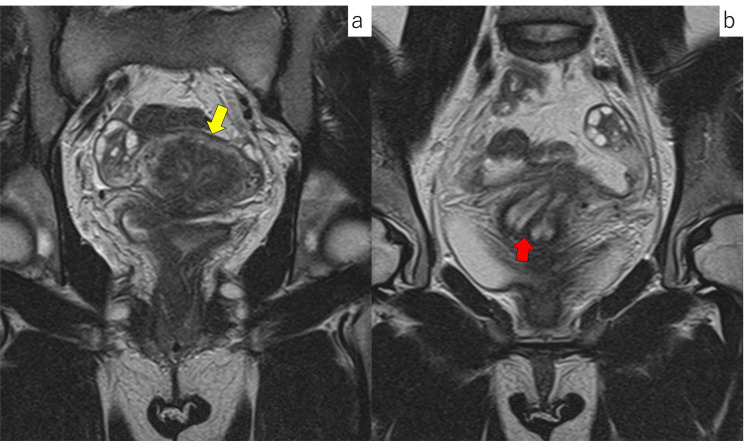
T2-weighted MRI images before pregnancy. (a) The appearance of the uterus revealed no indentation at the uterine fundus (yellow arrow), and the septum was separating the uterine cavity. (b) The septum extended to the cervix (red arrow). MRI: magnetic resonance imaging
